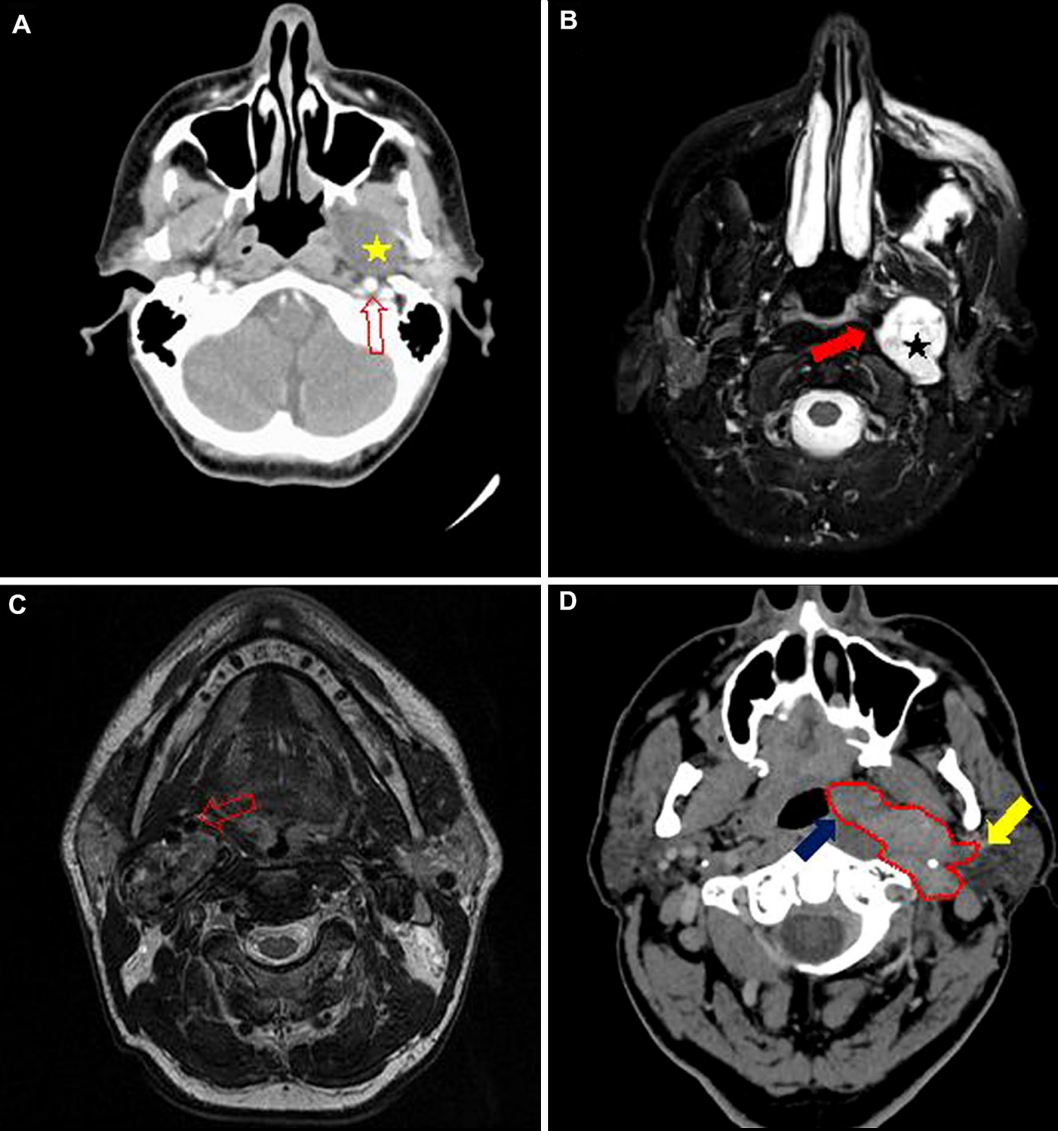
Radiological images of different parapharyngeal space tumors. a Axial contrast-enhanced computed tomography of a well-defined, minimally enhancing prestyloid tumor, a pleomorphic adenoma (asterisk) that posteriorly displaced the internal carotid artery (ICA) (arrow). b Axial T2-weighted magnetic resonance of a poststyloid space tumor, a vagal neuroma (asterisk) demonstrating homogeneous enhancement and anteromedial displacement of the ICA (arrow). c Axial T2-weighted magnetic resonance image of a tumor in the poststyloid compartment, a vagal paraganglioma that shows the typical “salt and pepper” sign (hypo- and hyperintense densities, relating to intratumor blood flow) and displaced the ICA anteriorly. d Axial contrast-enhanced computed tomography of an irregular tumor (red, dotted line) occupying both the pre- and poststyloid compartment, a squamous cell carcinoma that is weakly enhanced with contrast and presents no clear demarcation from either the lateral pharyngeal wall (blue arrow) or the parotid gland (yellow arrow)
